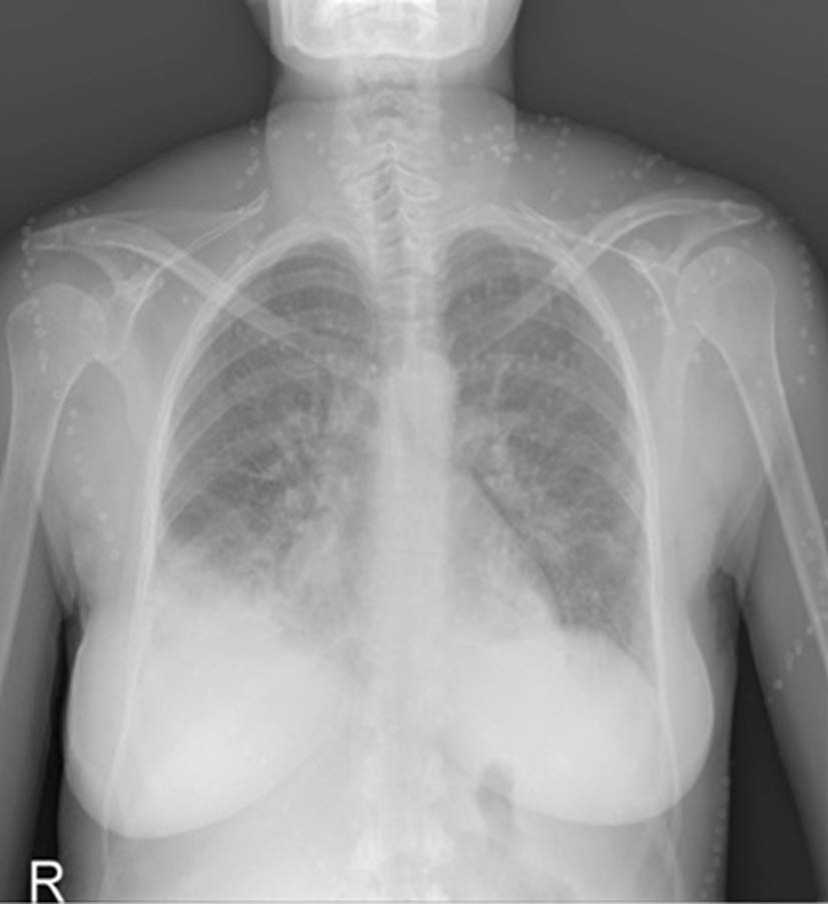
Anteroposterior chest radiography in a 36-year-old woman demonstrates right pleural effusion.
